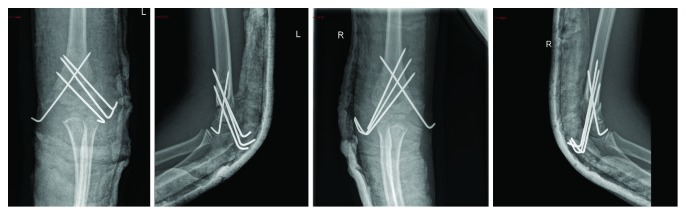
Day 1 post-op X-rays. AP and lateral views of the right and left elbows.
